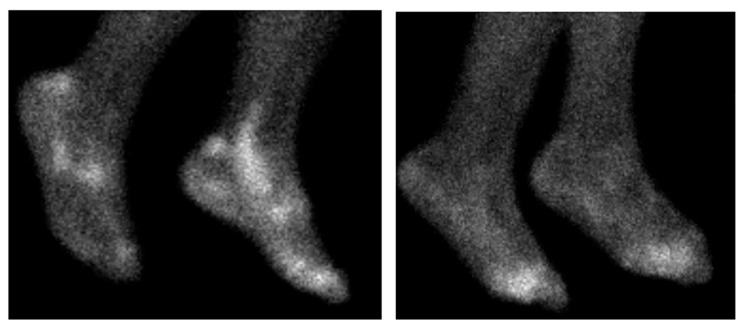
99mTc-Maraciclatide scintigraphy scans of the feet. The left showing a patient with psoriatic arthritis, with characteristic uptake within the achilles enthesis and peroneal tendons. The right showing a RA patient with metatarsophalangeal joint uptake.
